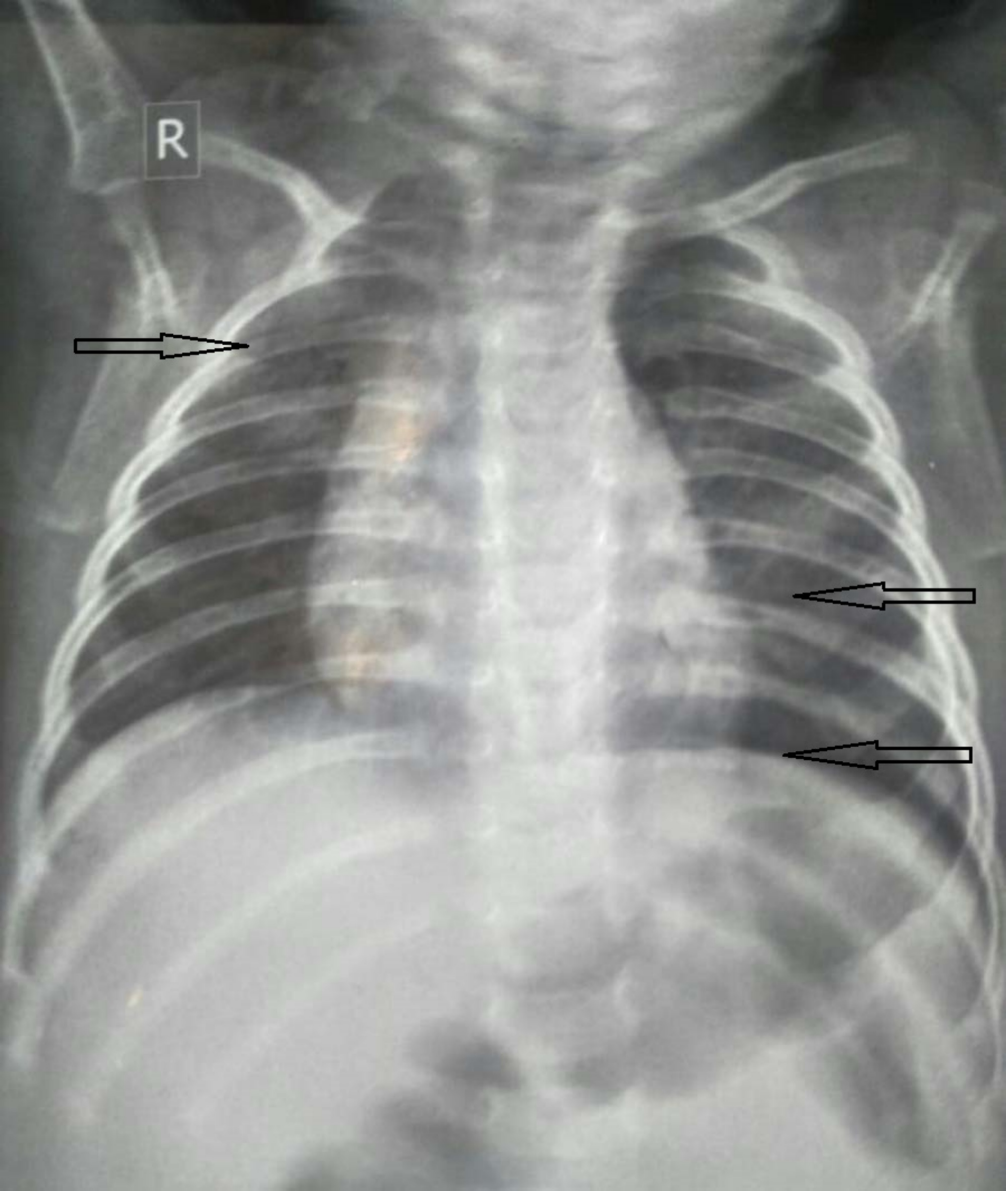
Chest X-ray representing hyperinflation of lungs with flattening of the diaphragm and increased hilar bronchial markings, suggestive of bronchiolitis.
